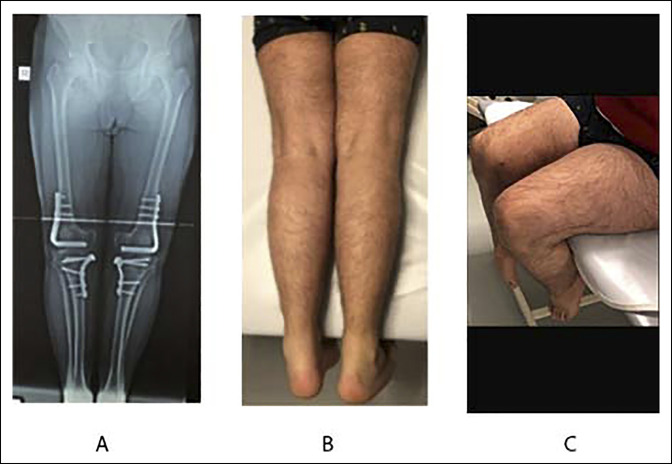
A, The anteroposterior radiograph showing the final standing long leg and (B–D) clinical and functional postoperative evaluation. The range of motion on the left knee was 0/0/125 and the range of motion on the right knee 0/0/120.
